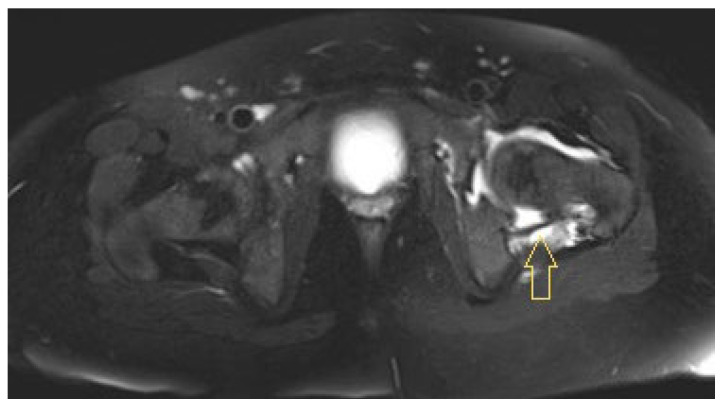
Initial magnetic resonance image of Patient 3: arthritis in the left hip joint and a reaction of the surrounding muscles. Arrow shows to the fluid path.
